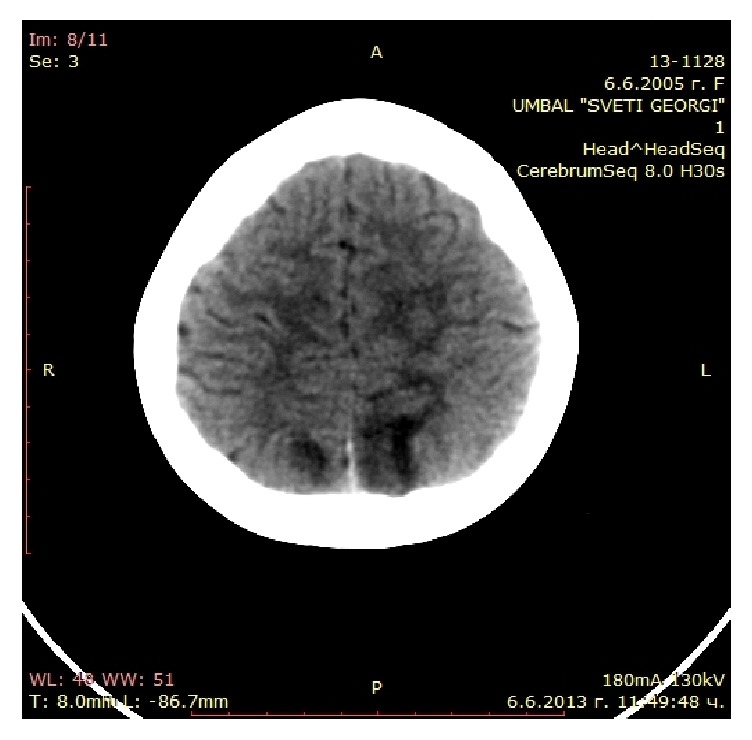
Head CT after the first episode with neurological symptoms: subcortical parietooccipital, parasagittal hypodense lesions in the white matter bilaterally but more on the left.
